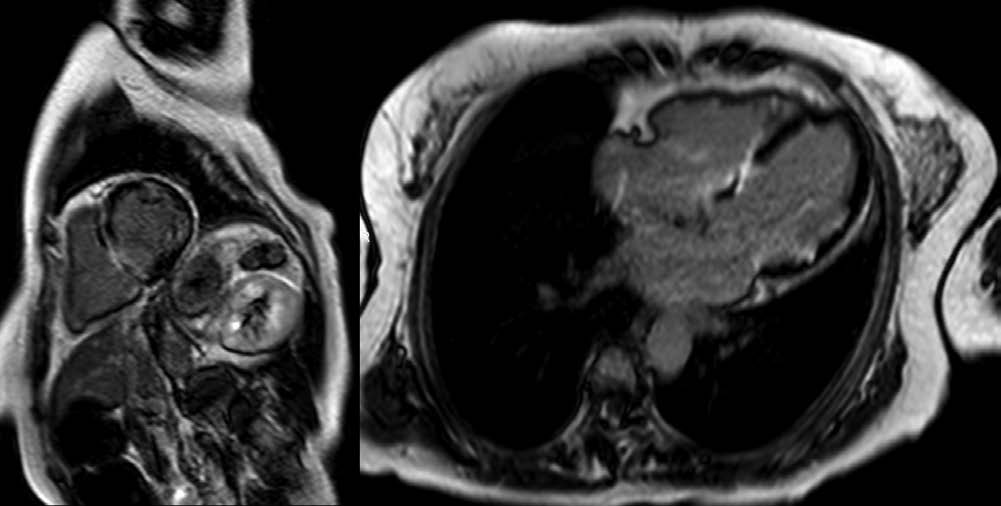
Cardiovascular magnetic resonance images. Cardiovascular magnetic resonance imaging revealed late gadolinium enhancement from the basal to midwalls of the left ventricle (left), as well as focal gadolinium enhancement on the midseptal and lateral walls (right).
